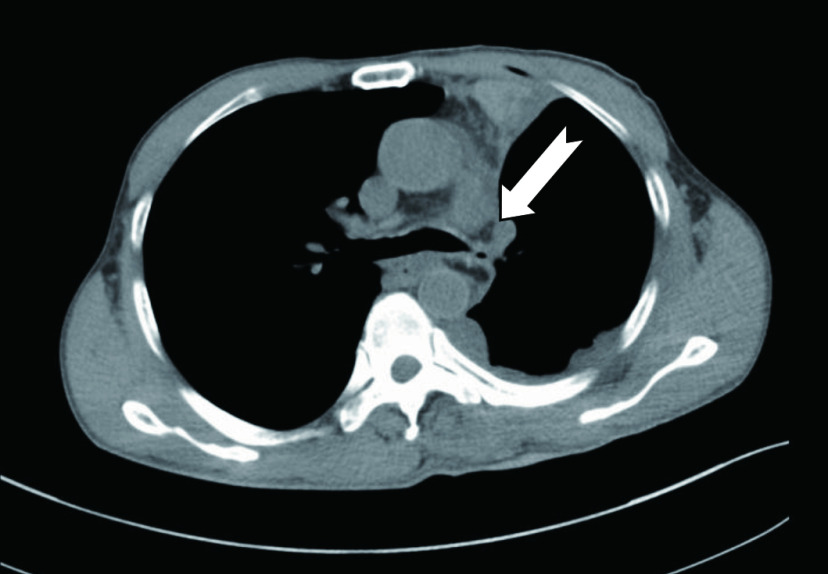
术后支气管吻合口及其周围的带蒂心包脂肪带的CT影像。白色箭头显示的脂肪密度组织为包裹在支气管吻合口周围的带蒂心包脂肪带 CT image of bronchial anastomosis and pedicled pericardial fat flap around it. White arrow showing pedicled pericardial fat flap wrapping bronchial anastomosis. CT: computed tomography
